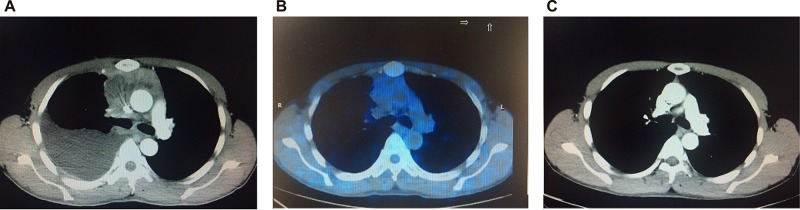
Imaging scans through the whole treatment for a patient of T-LBL Baseline CT scan (A) revealed a mediastinal mass and moderate pleural effusion. Interim PET-CT scan (B) showed positivity with residual lesion in the mediastinum. CT scan (C) after consolidation therapy confirmed CR.
